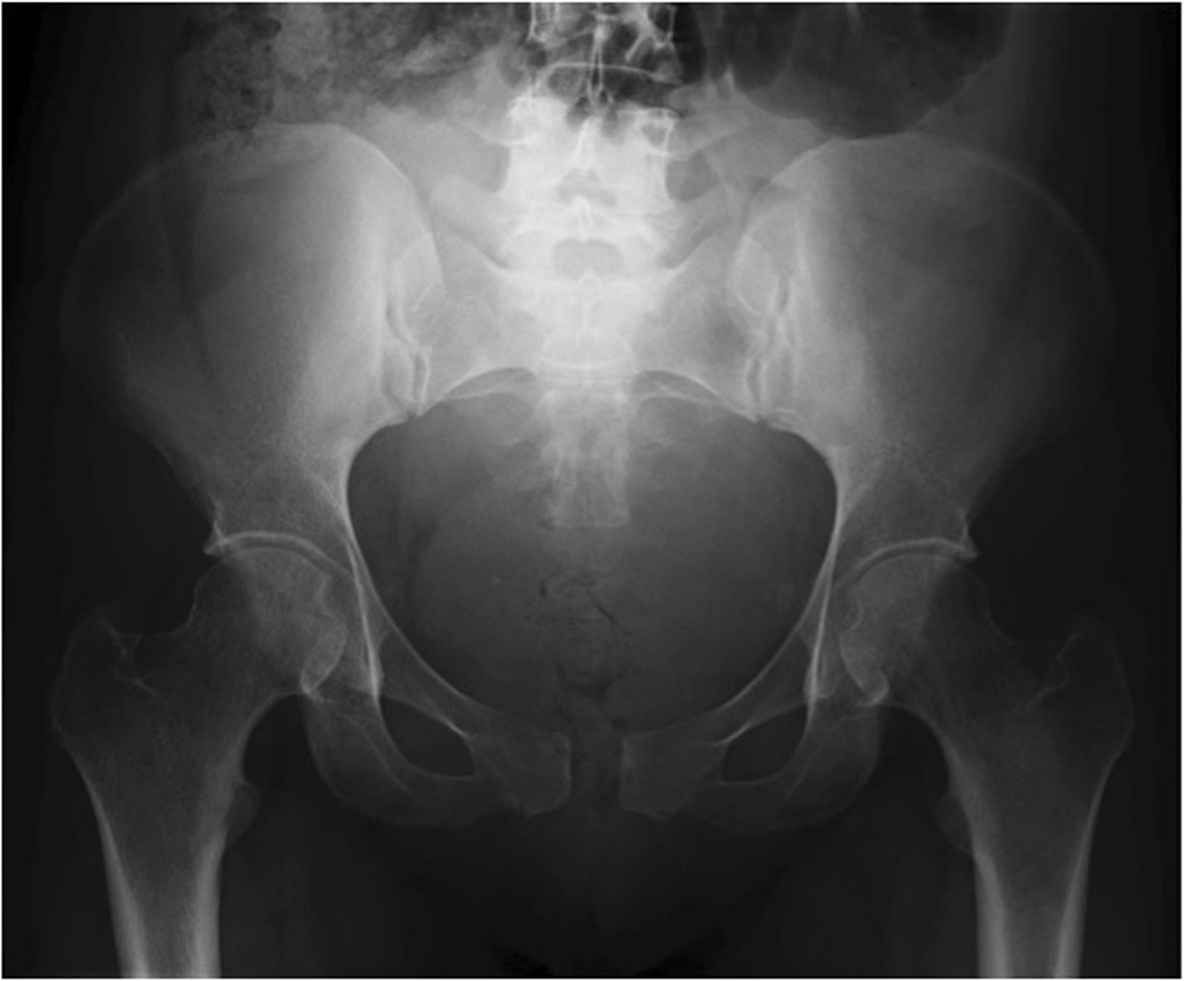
Plain X-ray of the pelvis performed at day 4. The antero-posterior pelvic radiograph reveals pubic symphysis diastasis and tiny irregularities of articular surfaces
